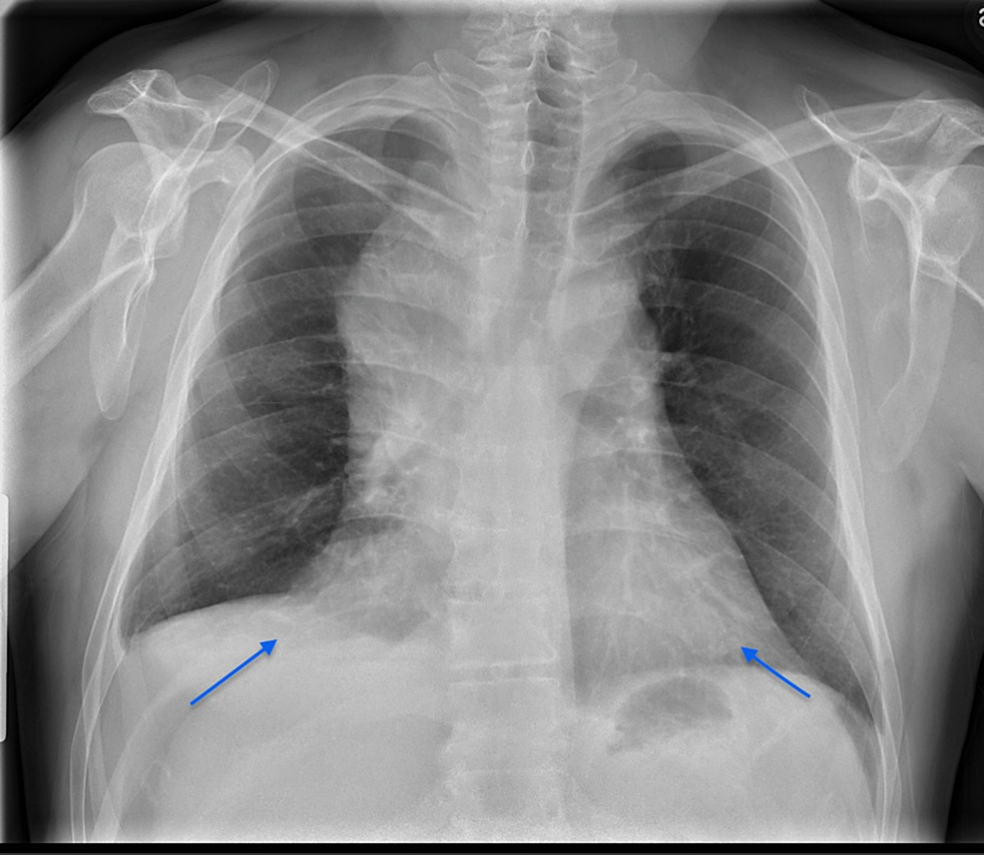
Chest X-ray showing bilaterally enlarged cardiac silhouette (arrows)
